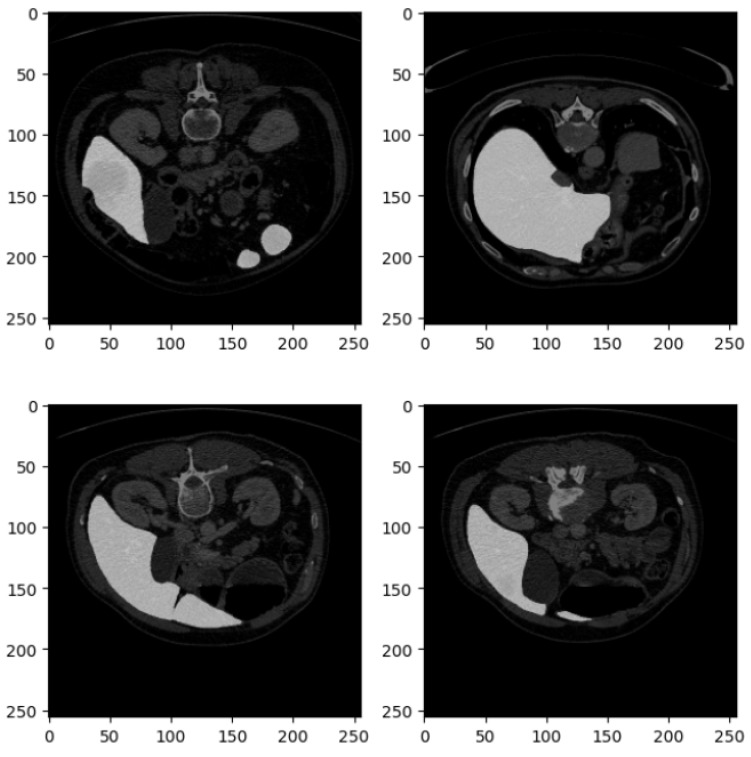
Four examples of CT images with the related mask obtained from the DII dataset.
